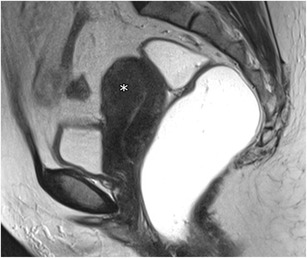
Postmenopausal uterus: Sagittal T2-weighted images; the junctional zone is not measurable (asterisk)
